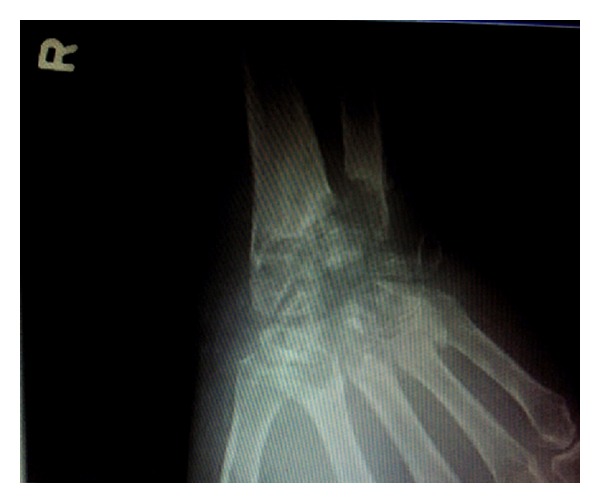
X-ray anteroposterior view of wrist joint: septic arthritis of distal radius/ulna, carpal bones, and bases of metacarpals.
